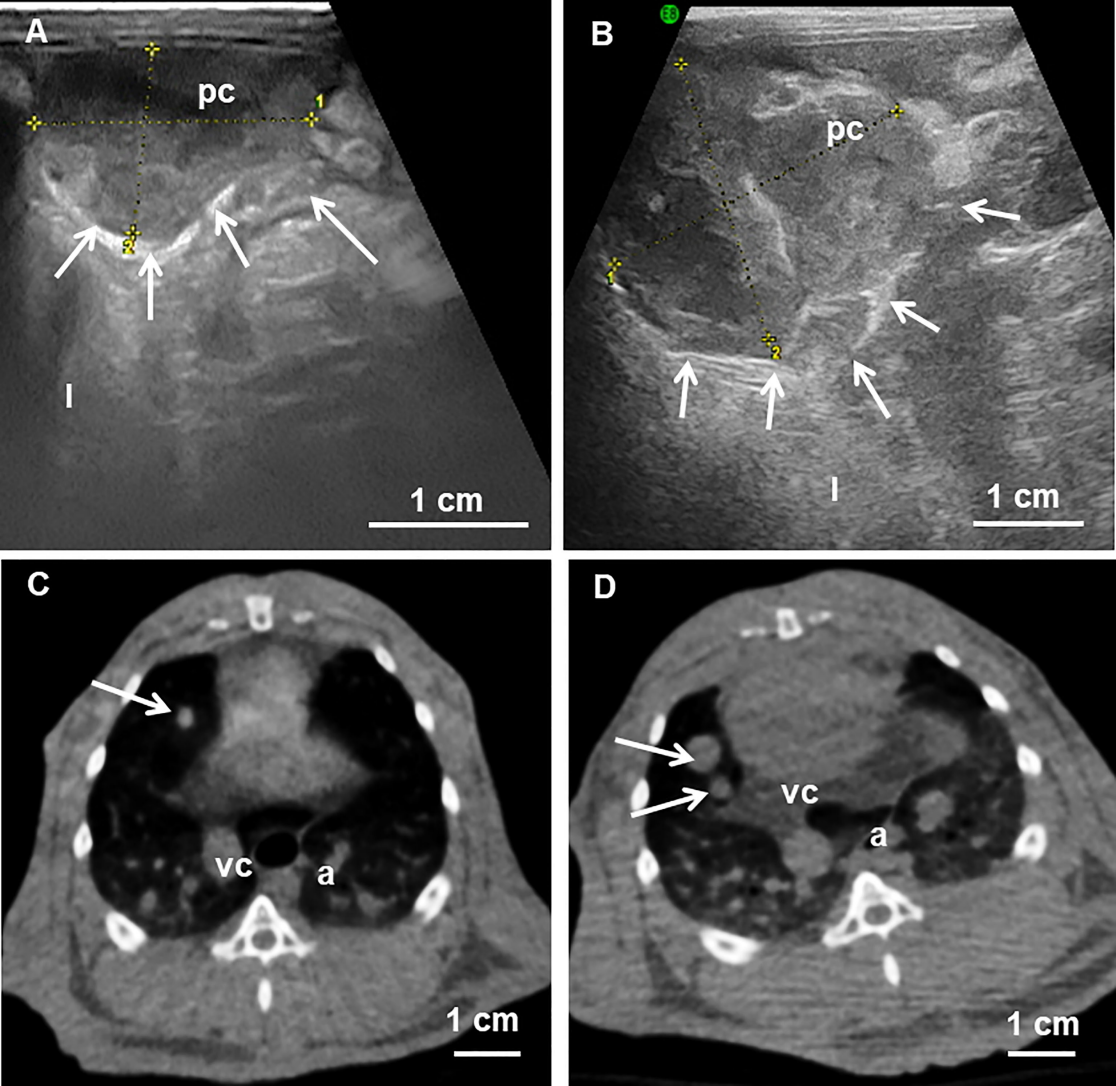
(A, B) Abdominal ultrasound examination 30 and 36 days after tumor inoculation showing abdominal peritoneal metastases and peritoneum surrounding the carcinosis (arrows). The metastases increased in size and became more invasive over time. (C, D) Cone beam CT at the thoracic level shows small pulmonary nodules (arrows) at 18 days that have increased in size one week later. pc, peritoneal carcinosis; l, liver; vc, vena cava; a, aorta. From (22).
